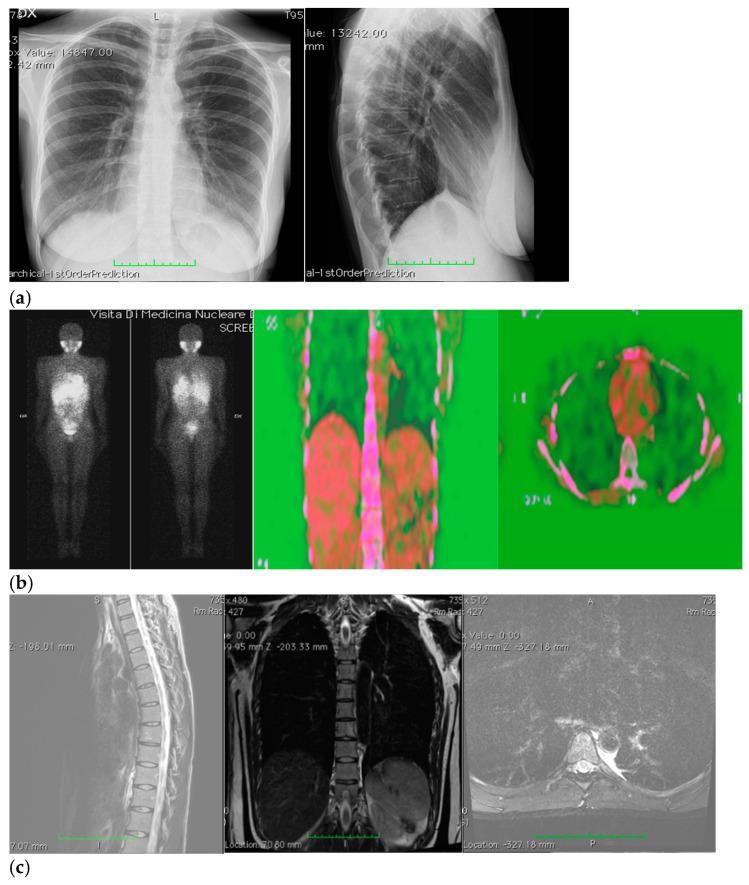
(a–c) Imaging assessments for post-surgical evaluation. (a) X-rays. (NOTE: No signs of ongoing pleuropulmonary lesions, free pulmonary bases, each hilum had a normal morphology, heart and aorta within normal limits, normal mediastinum). (b) Total-body scintigraphy with metaiodobenzylguanidine. (NOTE: No longer detectable pathological activity in the left hemithorax. No other areas of significant radiopharmaceutical were observed). (c) Thorax MRI with Gadoteridol. (NOTE: Outcomes of the surgical removal of the well-known left paravertebral lesion and a thin fluid layer corresponding to the surgical cavity. Very little slightly hyperintense remaining tissue in T2-weighted images, with subtle contrast enhancement and a maximum size of about 15 mm, at T6–T6 level, on the left side of the intervertebral foramen, close to the same level costovertebral joint—in a clinical picture which required MRI monitoring with contrast medium overtime).
